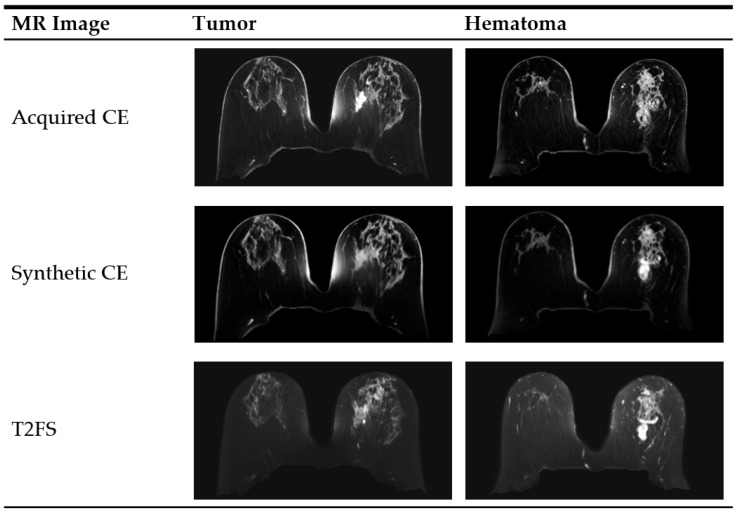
Example synthetic CE-MRI false-negative tumor volume. Axial-view of acquired CE-MRI, synthetic CE-MRI, and non-contrast acquired T2-weighted with fat suppression (T2FS) of tumor and hematoma slices of a patient with no overlap between acquired and synthetic tumor volume at pre-treatment (Dice coefficient = 0.0).
